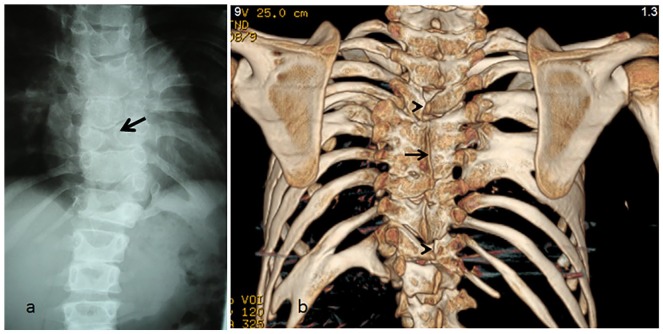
Loss of spine biomechanics. Anteroposterior spine radiograph in a 7-year-old child showed vertebral malsegmentation, that is, hemivertebrae and butterfly vertebrae (T6-11), respectively (arrow) (a) Three-dimensional (3D) reconstruction computed tomography (CT) scan of the spine and the thoracic cage in a 9-year-old boy with autosomal recessive Robinow syndrome presented with rigid spine movements (loss of the physiological spine biomechanics). He showed massive spondylothoracic malsegmentation associated with rib fusions and bifid ribs seen adjacent to the malsegmented spine segments. Strikingly, spina bifida occulta at the level of T3 and T10 (arrowheads) associated with a posterior malsegmented bar runs along T5-T8 on top of bilateral failure of segmentation. In addition to multilevel ribs fusions (right side, fusions of ribs 4 and 5, followed by fusions of 6, 7, and 8 ribs, respectively [arrow]). On the left side, fusions of ribs 6 and 7 followed by fusions of ribs 10 and 11 causing effectively the development of bifid rib (b).
